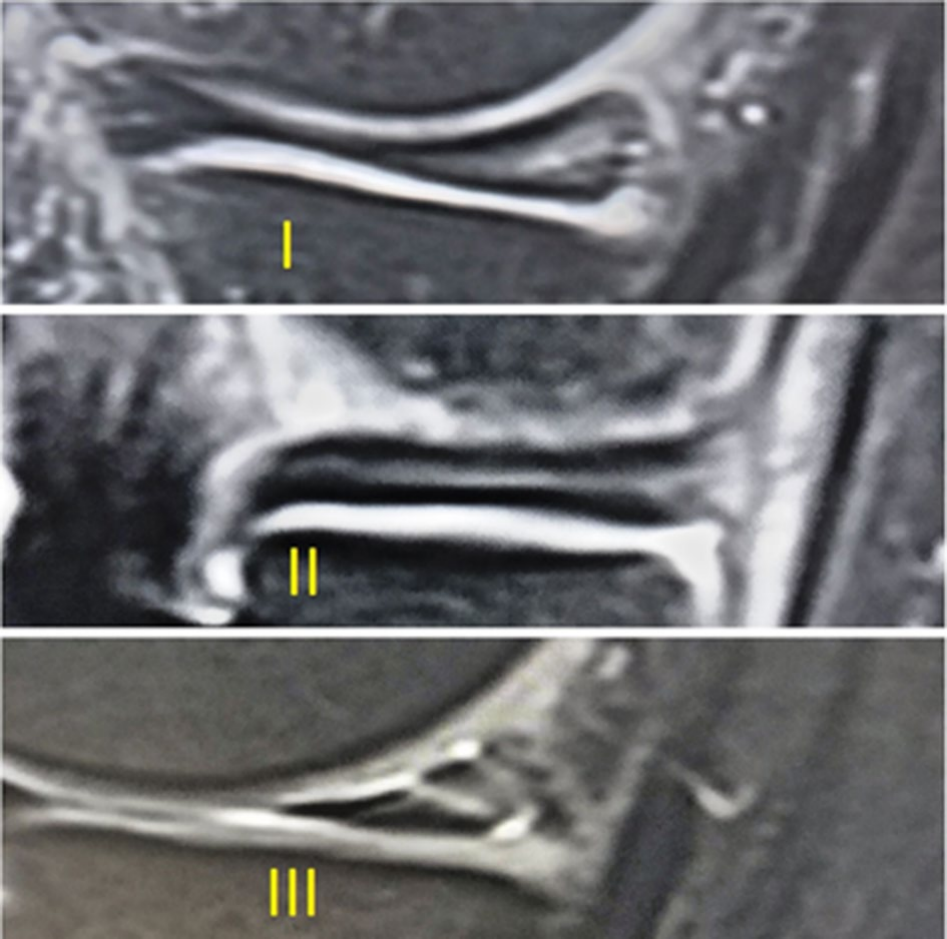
Meniscal changes grading scale on MRI.
